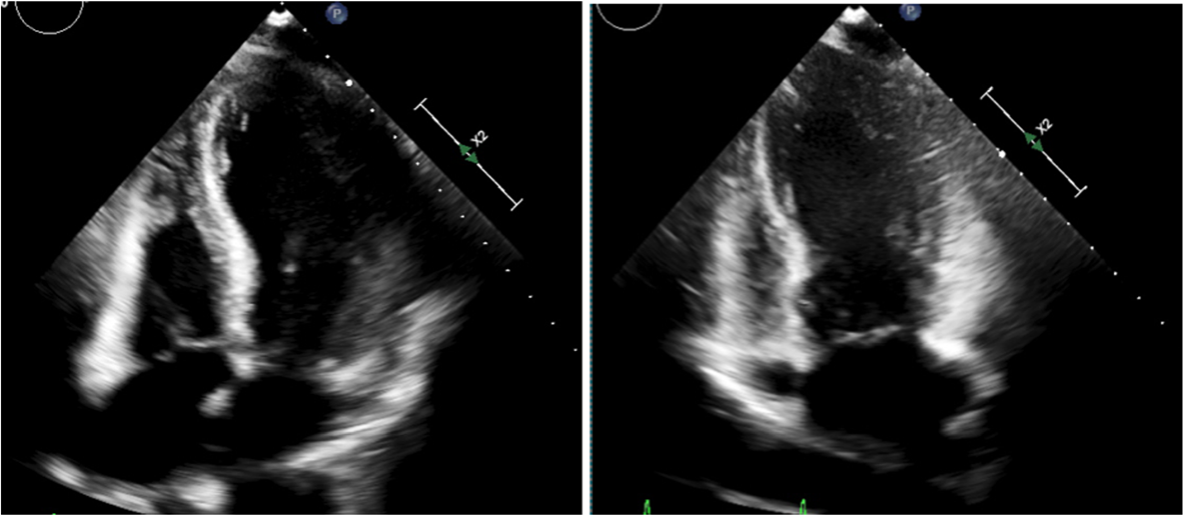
Echocardiogram of the patient showing hypokinetic apical and mid-distal walls and hyperdynamic basal walls of the left ventricle
